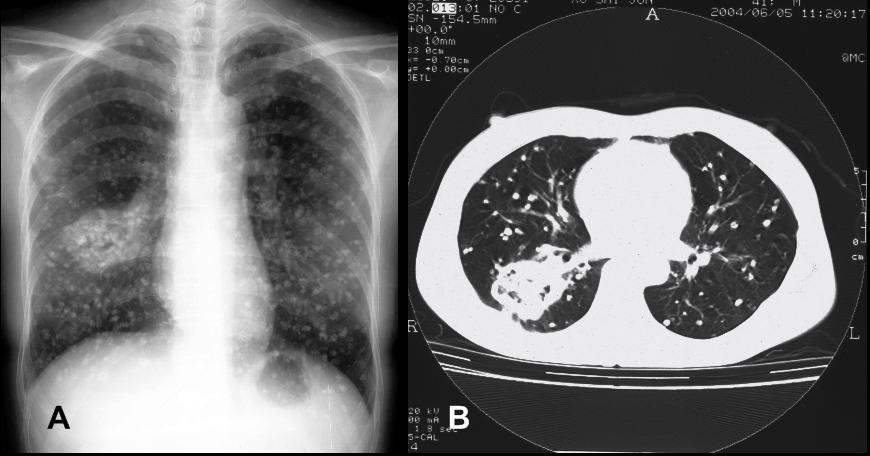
Radiographs of chest. Chest radiograph (A) and computerized tomograph (B) show an approximately 6.0 cm sized well-demarcated mass in the right lung and multiple calcified small nodules in both lungs.
